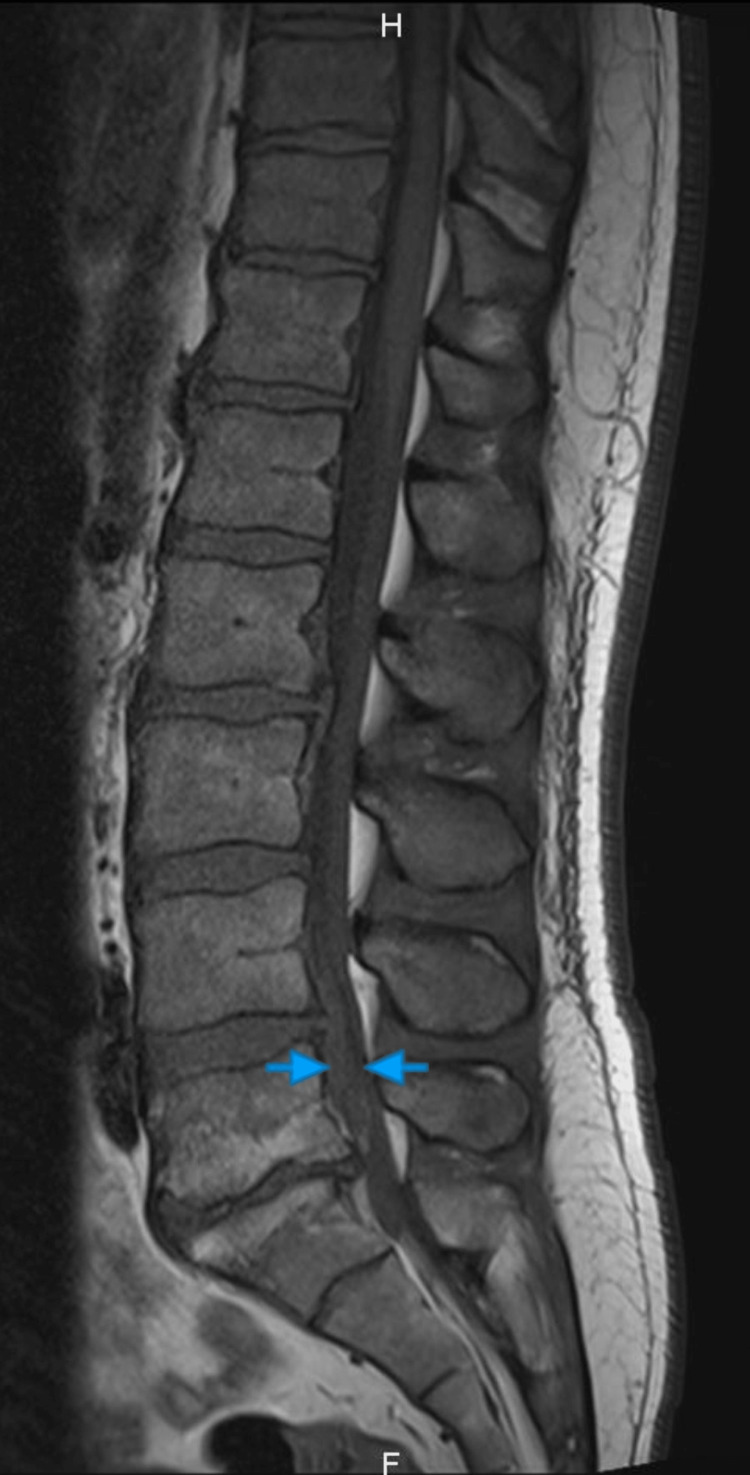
Sagittal T1-weighted image. Sagittal T1-weighted image showing spinal cord compression by an iso-intense structure at L4-L5 level, marked by two blue arrows on each side.
